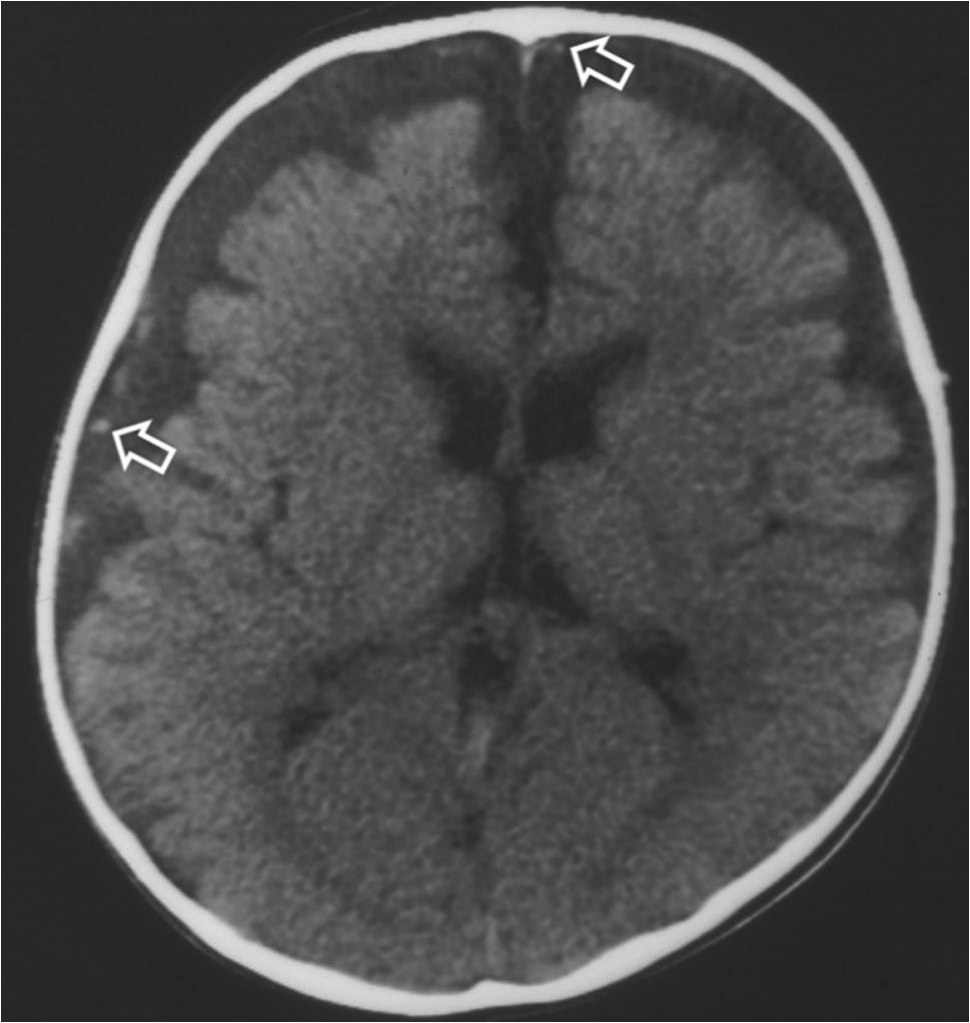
Axial brain computed tomography image of a 7-month-old boy with macrocrania and benign enlargement of the subarachnoid spaces. There is an enlargement of the subarachnoid spaces over the frontal lobes and at the interhemispheric fissure without cortical compression. Importantly, the vessels are located away from the cortical surface of the brain (*arrows*)
